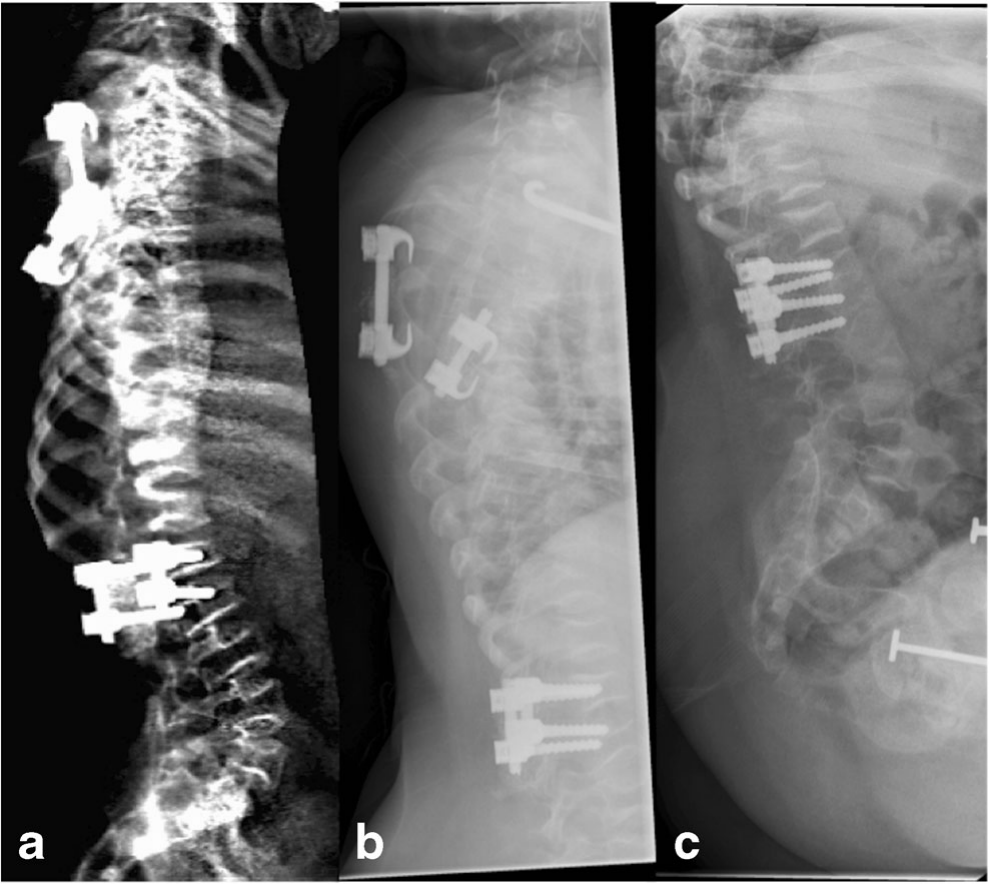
Lateral iDXA (a), thoracic spine radiograph (b) and lumbar spine radiograph (c) of patient 131, a 9-year-old female with osteogenesis imperfecta. The patient had severe multilevel fractures secondary to severe disease with resultant kyphoscoliosis degrading image quality on both iDXA and radiographs. On the consensus radiographic read T4 to T10 were graded as unreadable because of poor image quality
